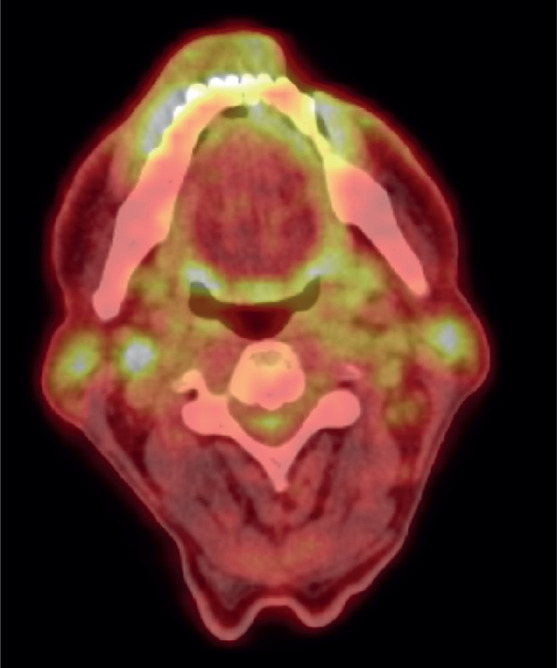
Post-chemotherapy PET CT scan, showing FDG avid residual left lower lip lesion.
